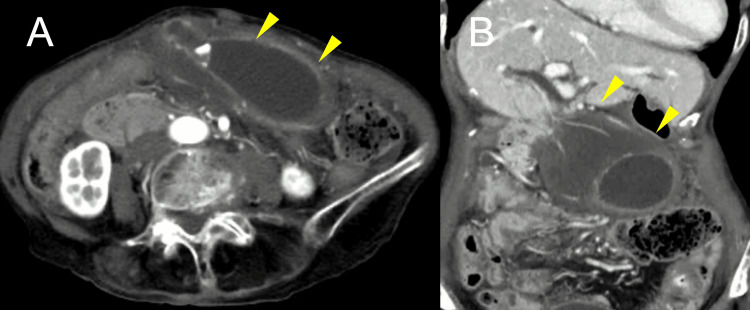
Radiological findings of gallbladder volvulus in Case 1 (A) Axial CT image shows medial displacement of the gallbladder with significant wall edema (yellow arrows). (B) Coronal CT image highlights the gallbladder’s medial displacement and edema (yellow arrows).
